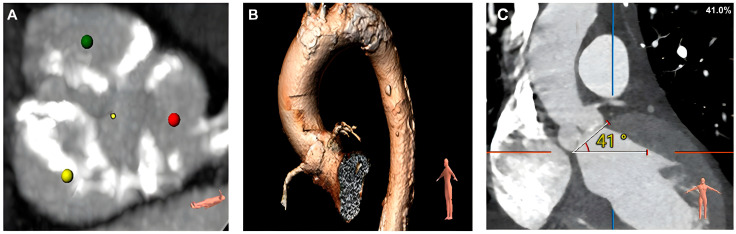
Calcified aortic leaflets (A) and mildly dilated ascending thoracic aorta with calcified plaques (B). (A) Detailed view of calcified aortic valve leaflets, highlighted by color markers (green, yellow, and red) that correspond to different regions of interest. Calcification is visible as dense areas in the images, indicating mineral deposits that reduce leaflet flexibility and affect valve function. (B) A three-dimensional reconstruction of the ascending thoracic aorta reveals mild dilation and the presence of calcified plaques along the vessel wall. The calcifications are depicted as dense, irregular structures within the aortic wall. (C) Computed tomography angiography demonstrating the angle between the aortic annulus plane and the axial plane (annular angulation) at 41°, suitable for TAVR.
